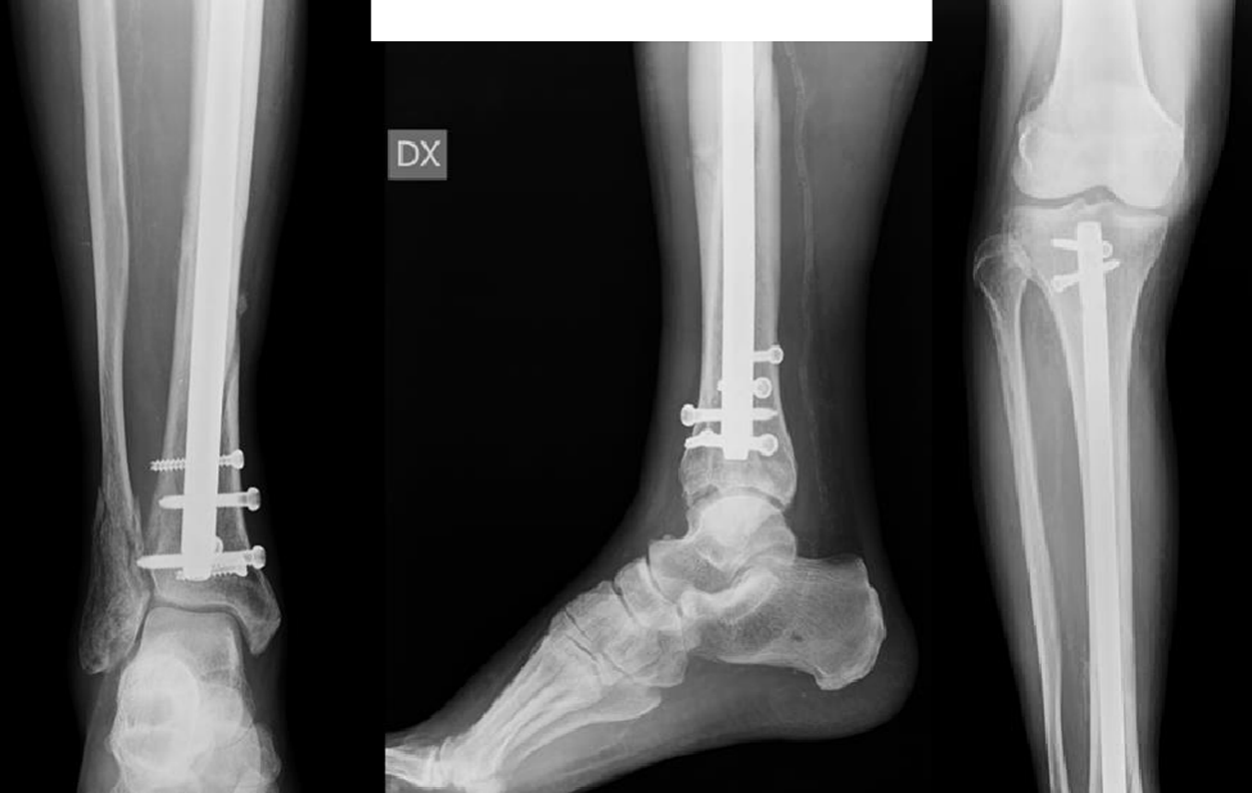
(a,b,c) Post-operative radiography at 3 months follow-up of IMN.
